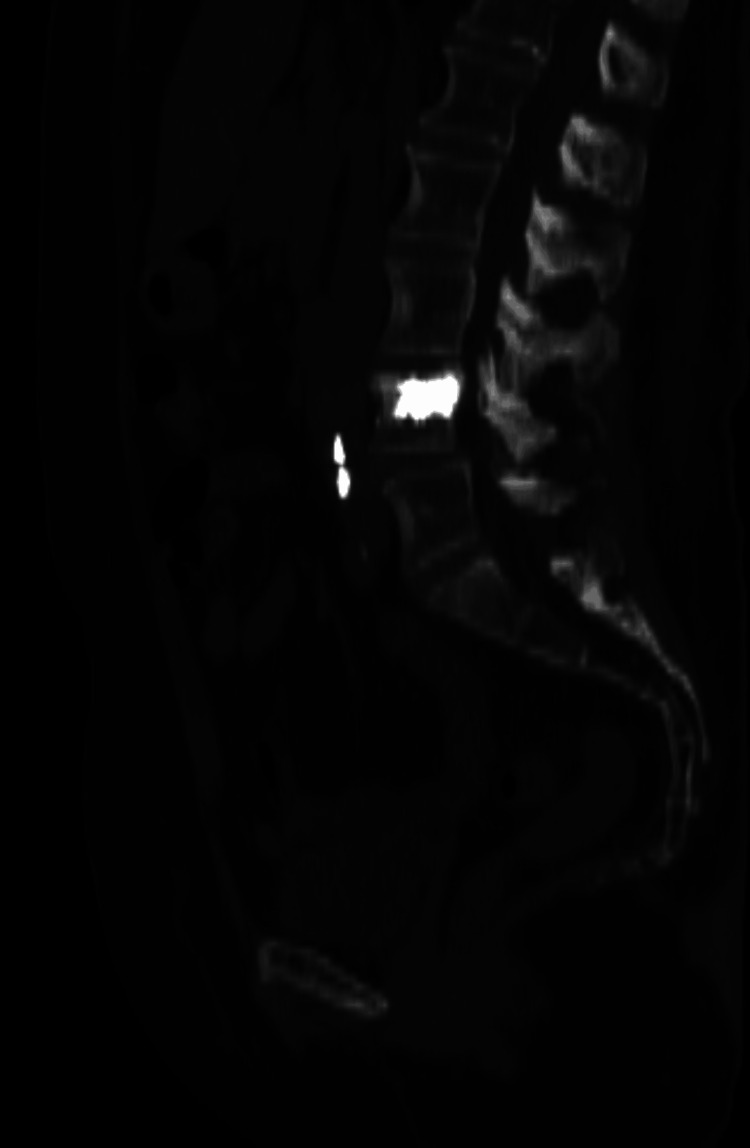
CT scan of the abdomen (sagittal cut) showing hyperdense emboli in the adjacent venous system with the same density as the injected material in the repaired vertebra CT: computed tomography
